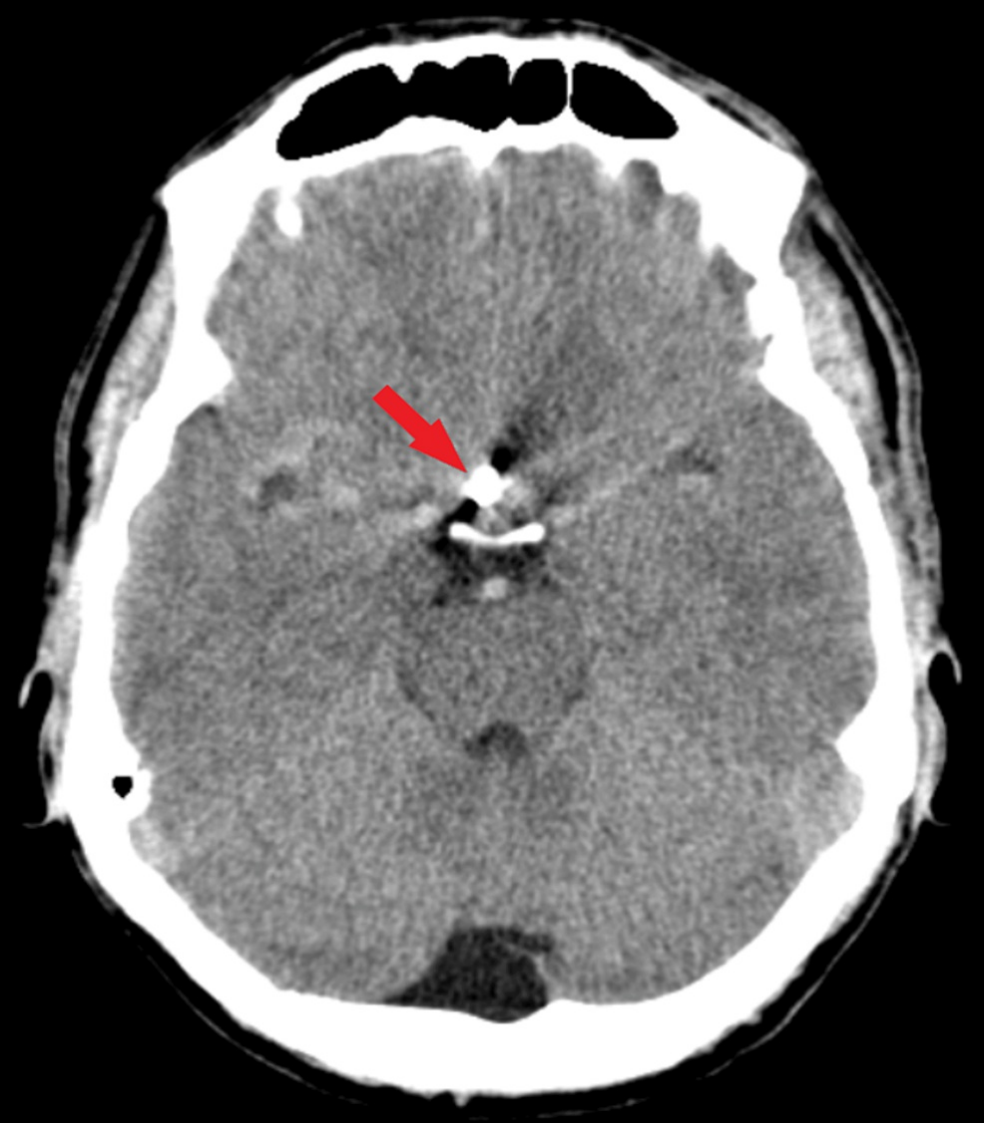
Postoperative CT scan. Axial section of CT scan tissular window demonstrate correct clip positioning (red arrow), without active hemorrhage.
